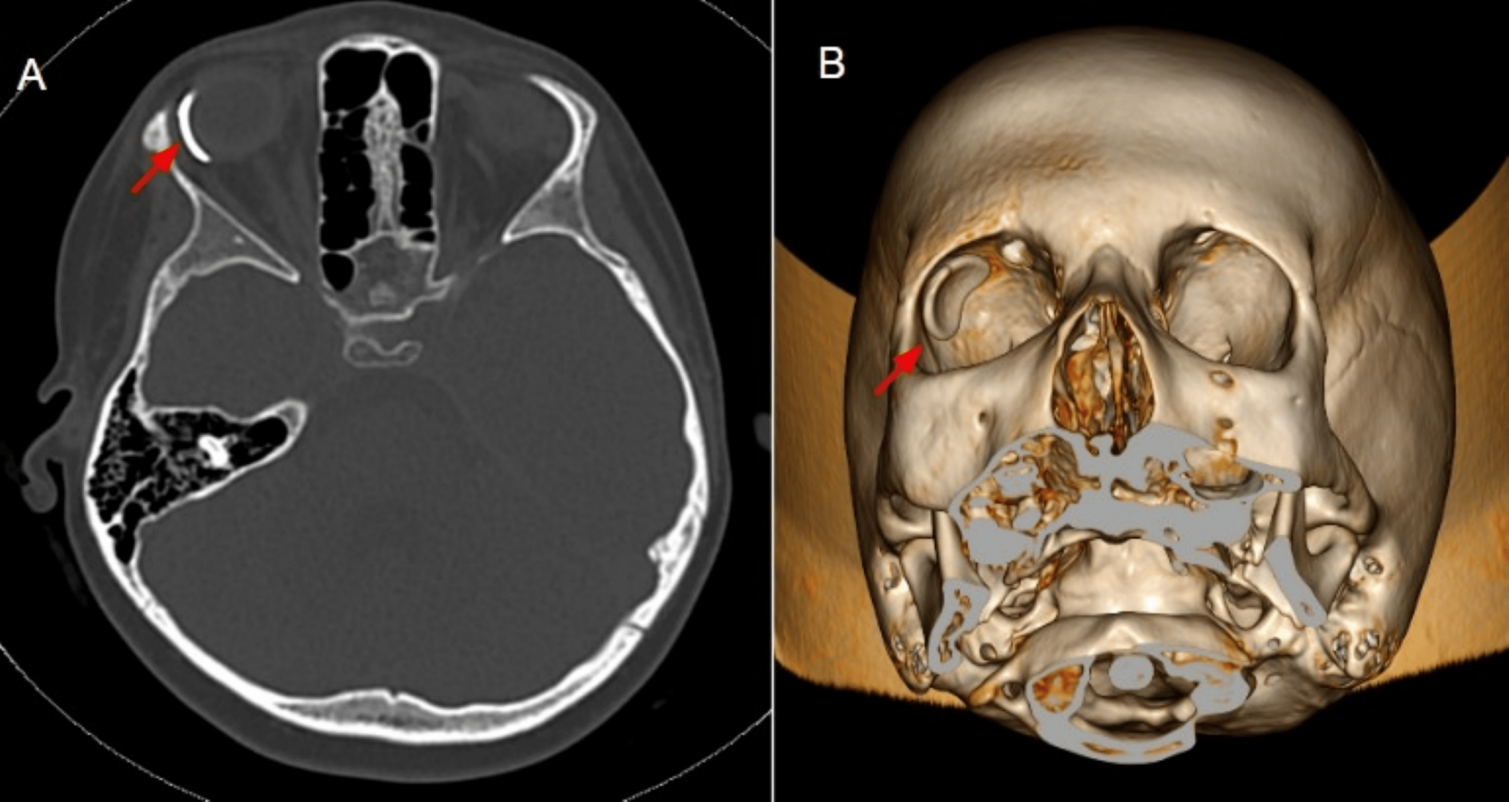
Axial CT bone window image Axial CT bone window image (A) and 3D reconstruction (B) demonstrate right eye Baerveldt tube implantation (red arrow) for glaucoma management, performed at seven years of age.
